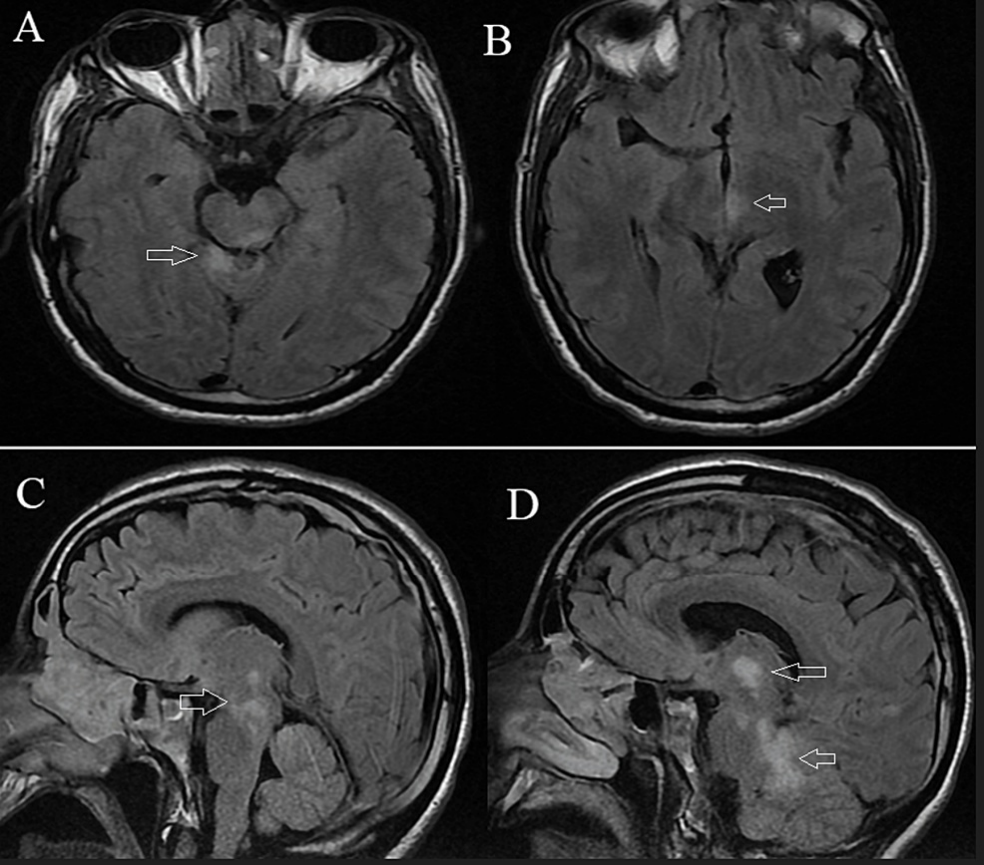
Cerebral magnetic resonance imaging on axial fluid-attenuated inversion recovery sequence demonstrating lesion in the cerebellar vermis (A) and left thalamus (B) (arrows). The sagittal section identifies extensive lesions of the meso-diencephalic region (C and D) (arrows).
